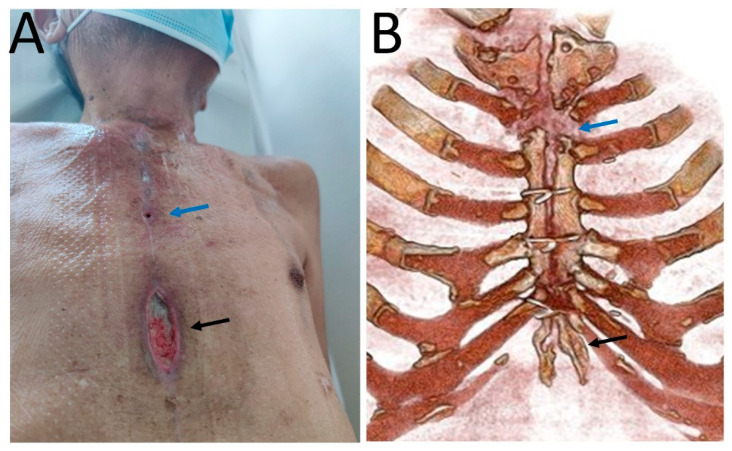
(A). Clinical picture of the two dehiscences in the upper and lower third of the sternotomy scar (arrows); (B). Three-dimensional volume-rendered CT images show sternal bone rarefaction areas in the upper and lower third of the sternum (arrows).
